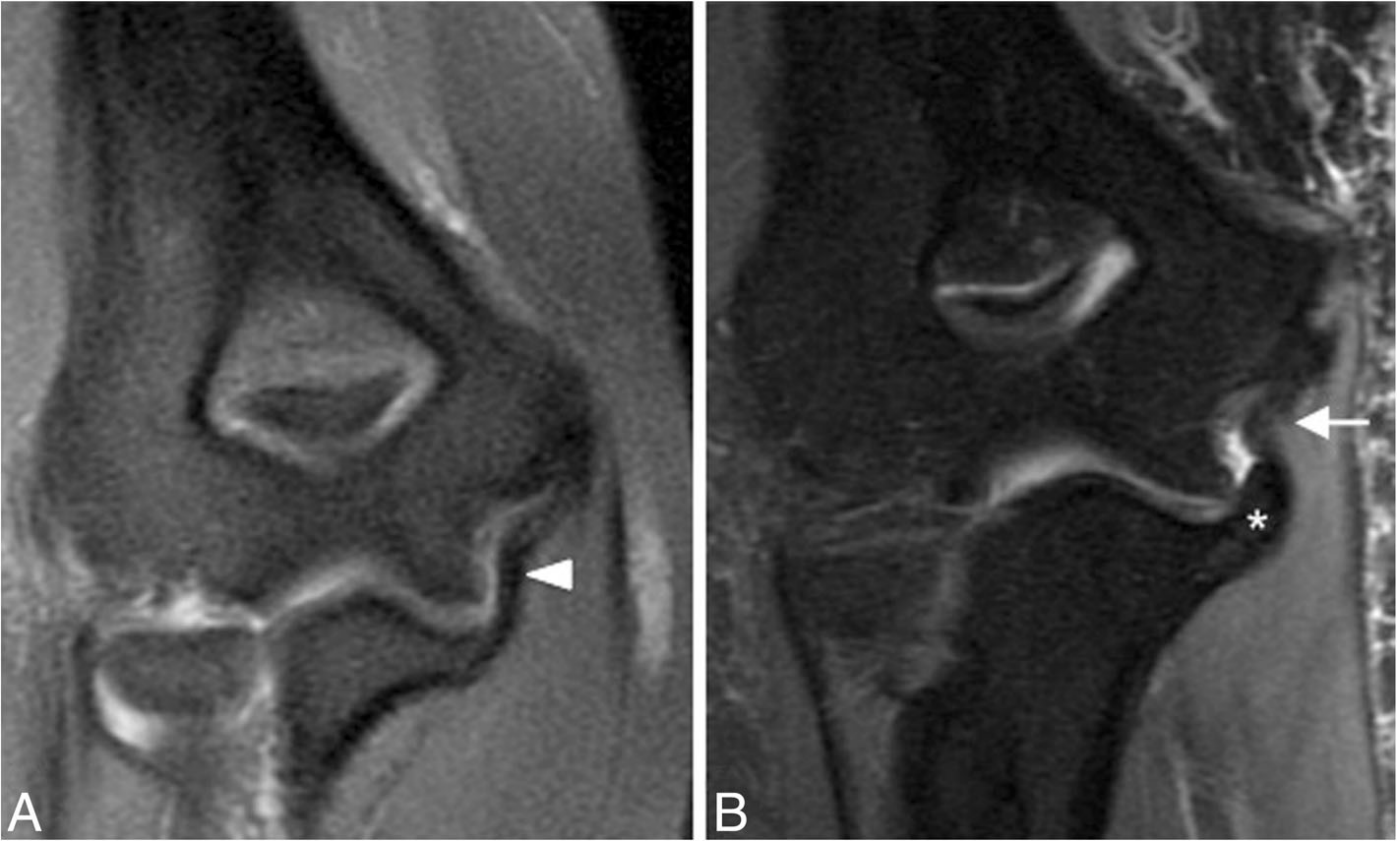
Two different patients with chronic elbow pain and medial instability. Coronal FS PD-weighted MRI (a) showing a chronically thickened anterior bundle of the medial collateral ligament (white arrowhead). Coronal FS PD-weighted MRI (b) showing a chronically thickened anterior bundle of the medial collateral ligament (white arrow), and a deformity of the sublime tubercle due to malunion of an old fracture (white asterisk)
